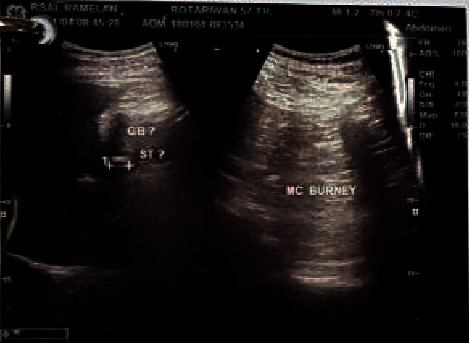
Abdominal ultrasound: normal gall bladder with stones without dilatation of the bile duct.
